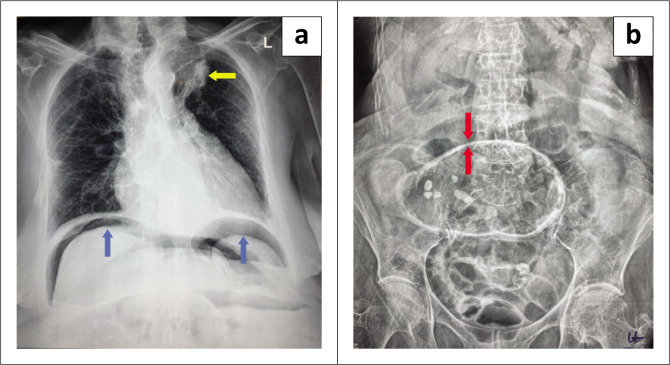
(a) Erect chest radiograph demonstrating free subcostal air (blue arrows) and a left paratracheal opacity causing right tracheal deviation (yellow arrow). (b) Supine abdominal radiograph demonstrating pneumoperitoneum, Rigler’s sign (red arrow).
